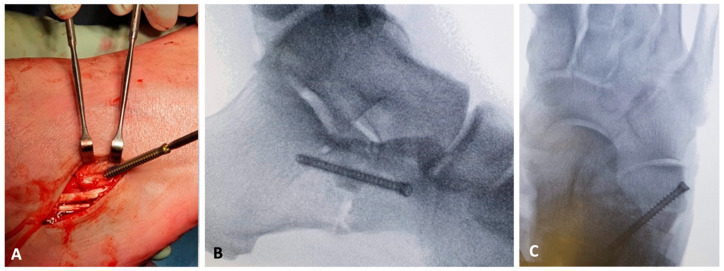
Lateral calcaneal lengthening osteotomy (LCLOT): fixation method. The graft is held in position with a screw (in this case with a CCS 4.0 mm screw, Medartis, Basel, Switzerland) (A). Dorsoplantar (B) and lateral (C) X-ray views show the correct position of the screw, the graft, and the bone.
